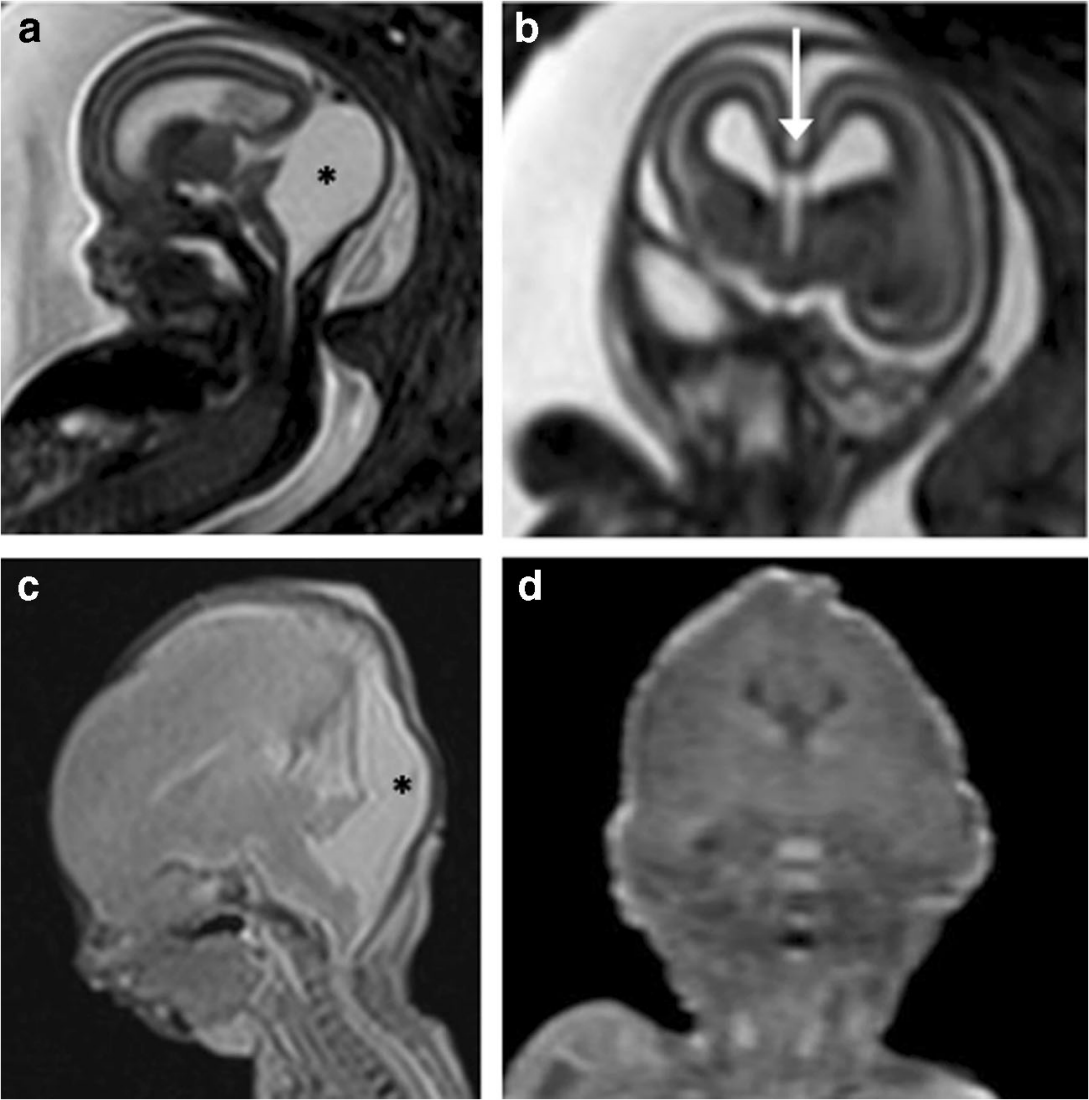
Dandy−Walker malformation (DWM) with callosal agenesis in a 19-week gestation fetus. a Sagittal iuMR showing upwardly rotated cerebellar vermis with large retrocerebellar CSF space (asterisk) enlarging the posterior fossa, consistent with an enlarged Blake’s pouch cyst. b Coronal T2-weighted iuMR demonstrates a thinned corpus callosum (white arrow) at the base of the anterior hemispheric fissure. c Sagittal T2-weighted and d coronal T1-weighted PMMR images were interpreted as a Dandy–Walker malformation with agenesis of the corpus callosum given the ‘steer-horn’ appearances of the lateral ventricles on the coronal view and posterior fossa cyst (asterisk)
